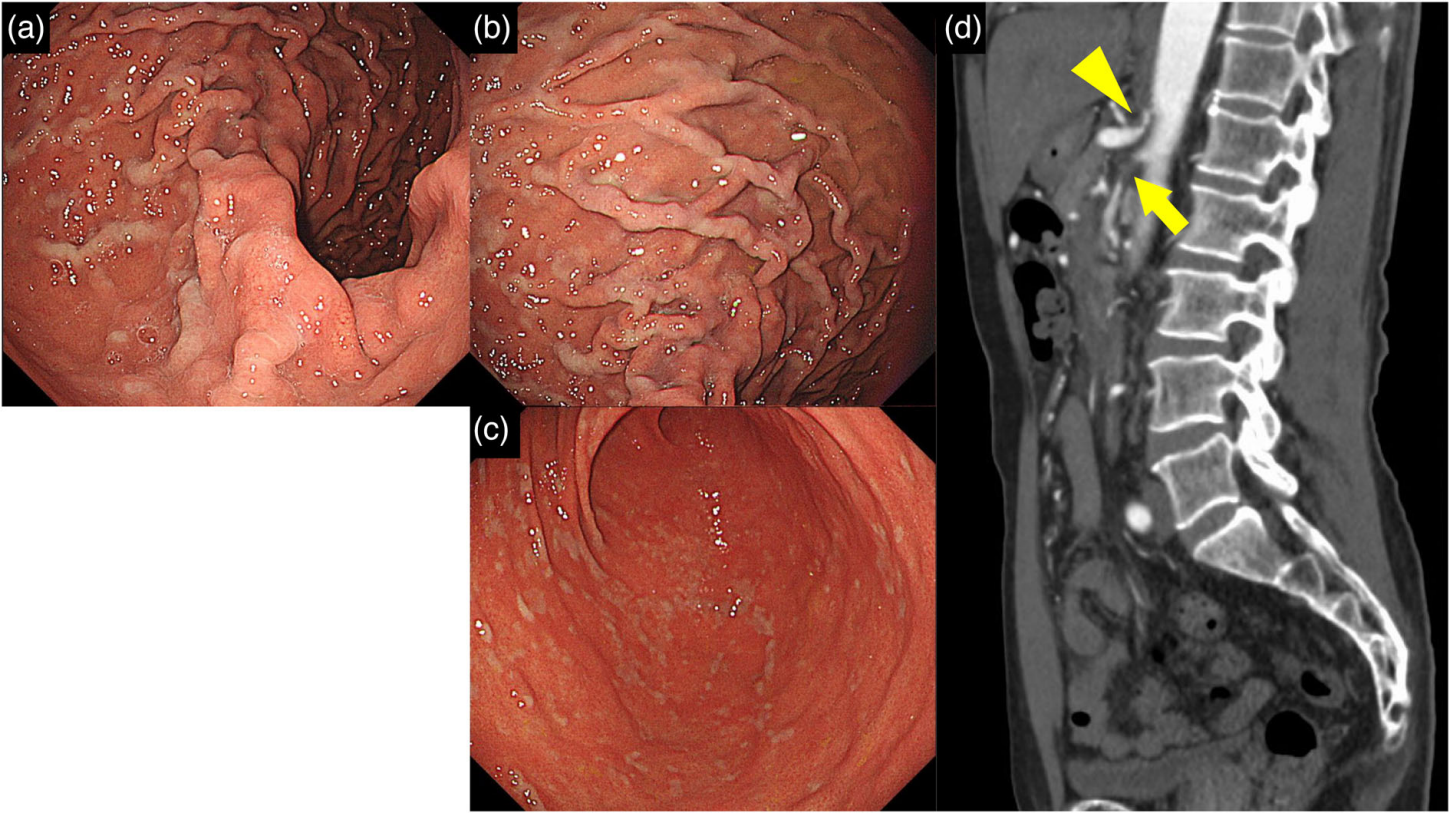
Endoscopic and Angiographic computed tomography findings at diagnosis. GIF‐H290Z was used for observation. (a) The posterior wall of the upper stomach body. (b) Fundus. (c) Antrum. Endoscopic findings after gastric dilatation. The mucosa was white in patches. (d) Angiographic computed tomography images. The superior mesenteric artery was occluded but was nourished by collateral circulation (arrow). The celiac artery was seen to be narrowed and dilated its periphery (arrowhead)
